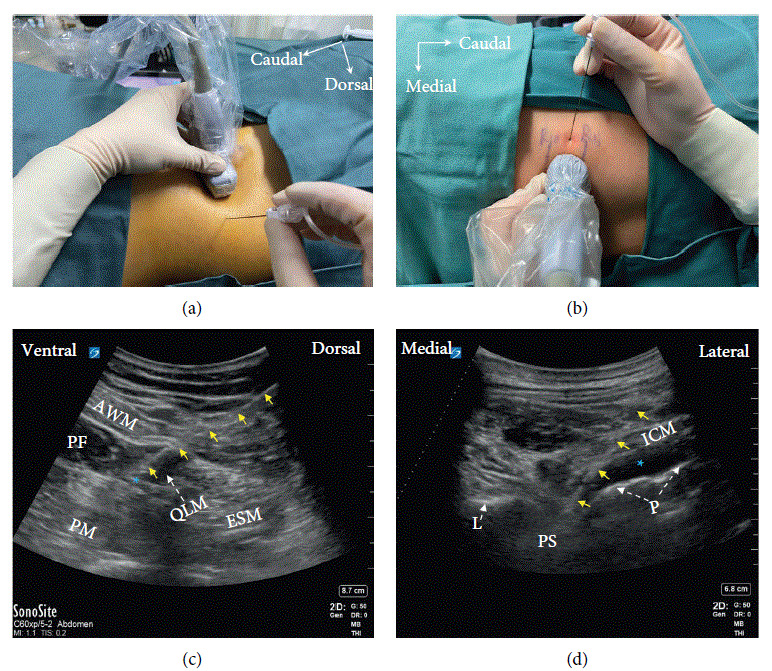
Ultrasound-guided transmuscular quadratus lumborum block (a, b) and thoracic paravertebral block (c, d). AWM: abdominal wall muscle, EPF: extraperitoneal fat, ESM: erector spine muscle, PM: psoas muscle, QLM: quadratus lumborum muscle, P: pleura, PS: paravertebral space, L: lamina, ICM: intercostal muscle, ^*∗*^: LA, yellow arrows: needle.
